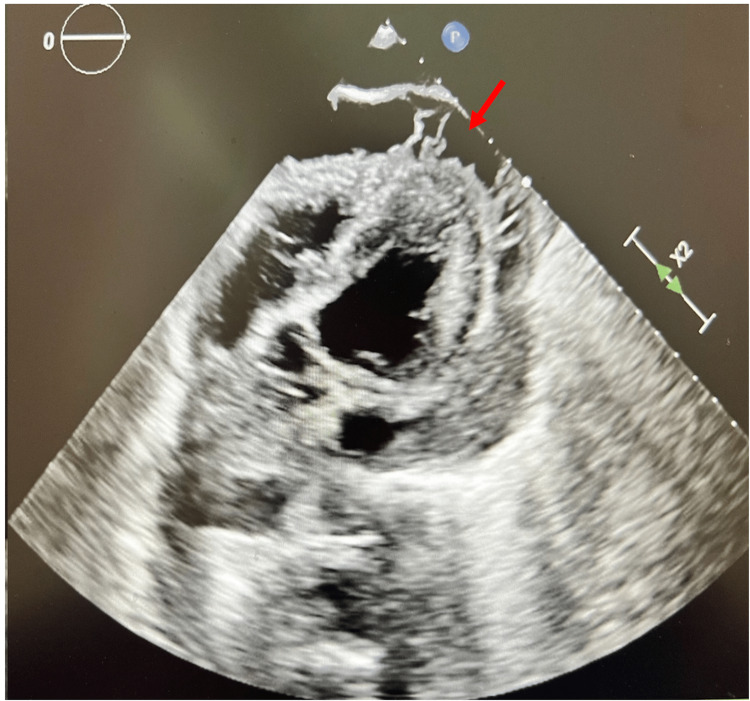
Immediate post-code bedside transthoracic echocardiogram showing a pericardial effusion (red arrow)
